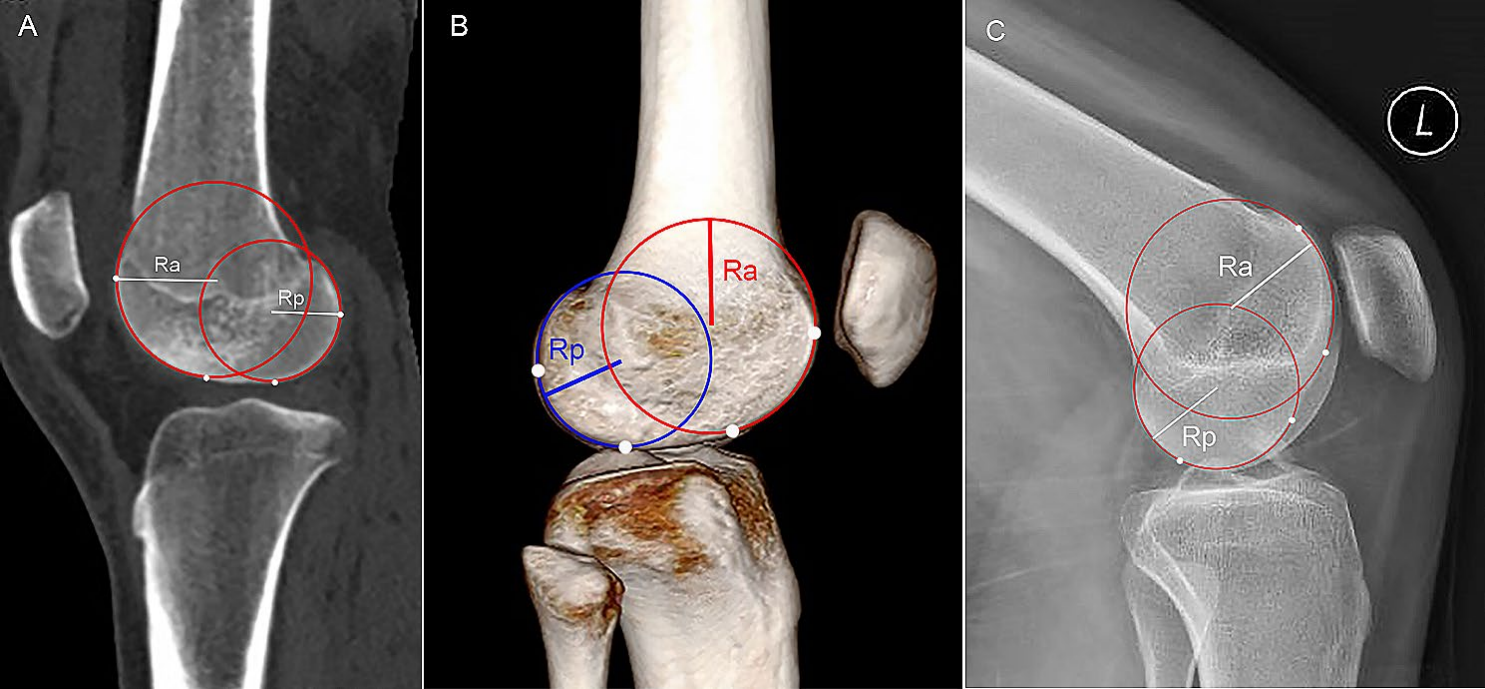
The measurement of lateral femoral condyle ratio (LFCR) by radiological data. (A) The midsagittal CT slice showing intact anterior and posterior femoral condyles is selected. (B) 3D–CT is reconstructed and standard lateral view is selected. (C) The lateral view of conventional radiograph is selected. Two circles are drawn to the bony edge and are congruent with the sphericity of the lateral femoral condyle. LFCI is calculated by a formula: LFCI = Rp/Ra
